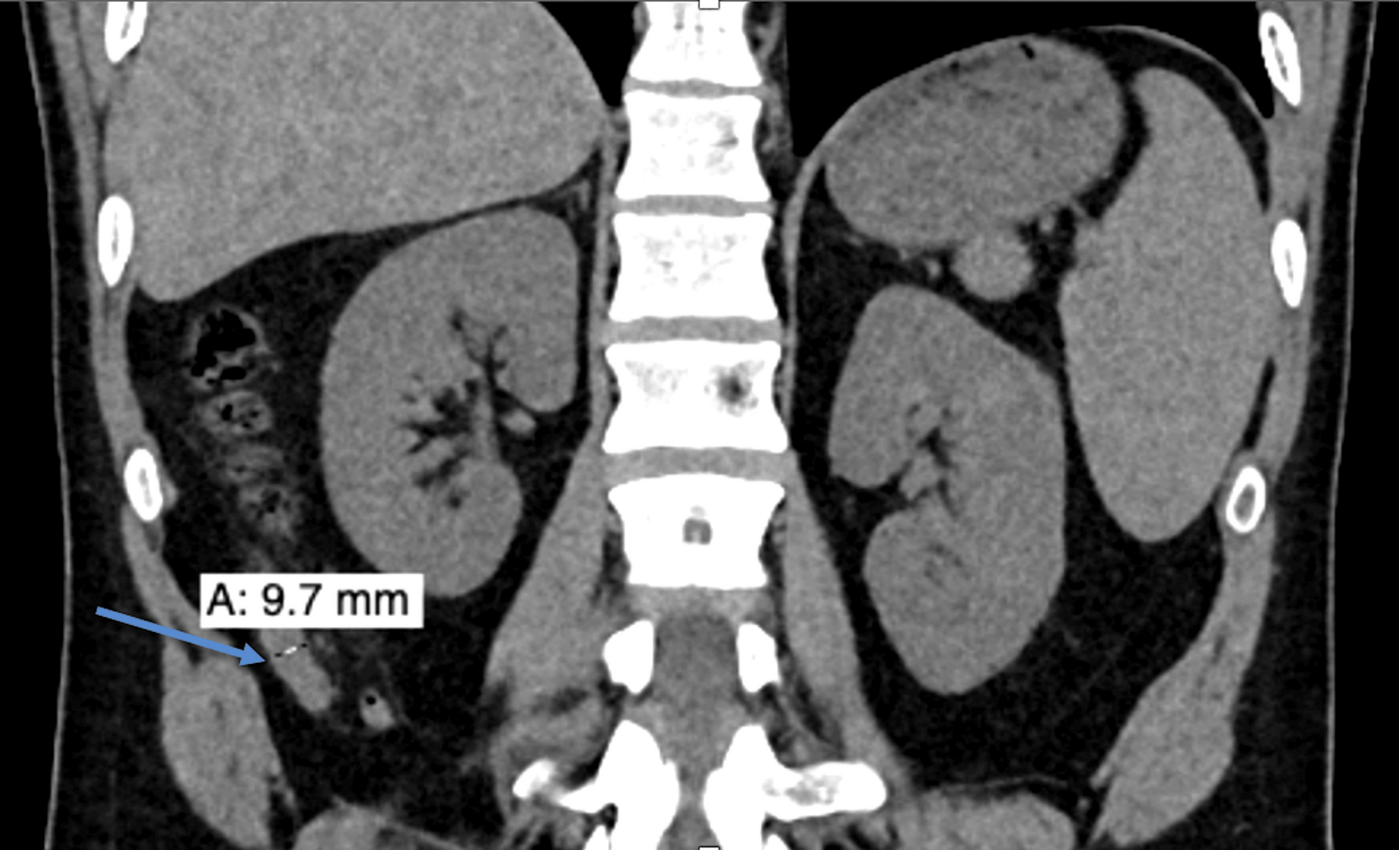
Coronal non-contrast computed tomography (CT) scan of the abdomen showing an enlarged appendix (arrow) Coronal view of a non-contrast CT scan of the abdomen demonstrating an enlarged appendix measuring 9.7 mm in maximal diameter, located in the right lower quadrant. Periappendiceal fat stranding is present, consistent with early acute appendicitis. The arrow indicates the location of the enlarged appendix.
